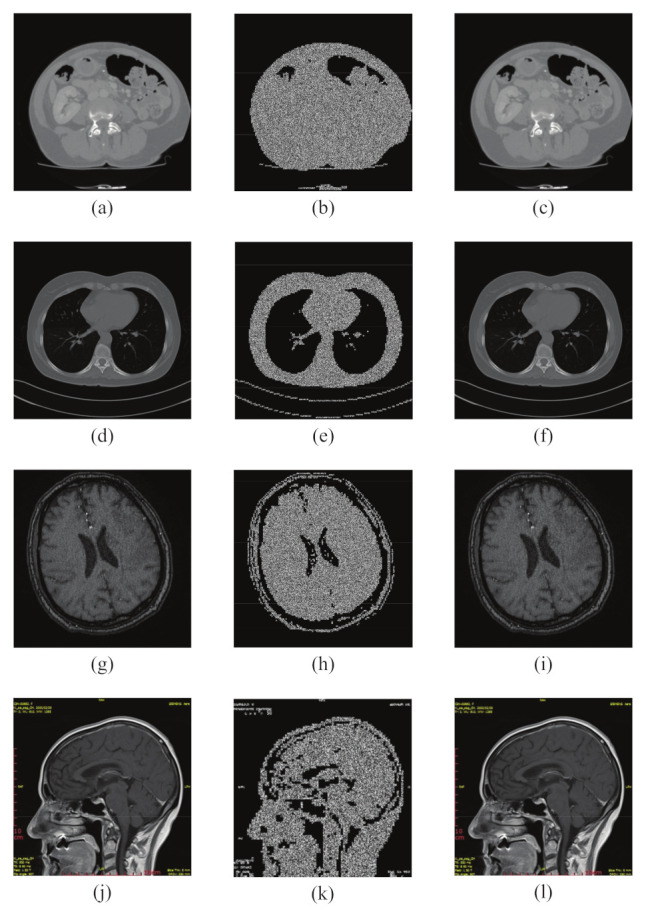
Encryption/decryption results: (a,d,g,j) show the plain images of four different structures, (a) CT Abdomen, (d) CT Lung, (g) CT Brain, and (j) MRI Brain. (b,e,h,k) are the corresponding ciphertext images. (c,f,i,l) are the decrypted images.
